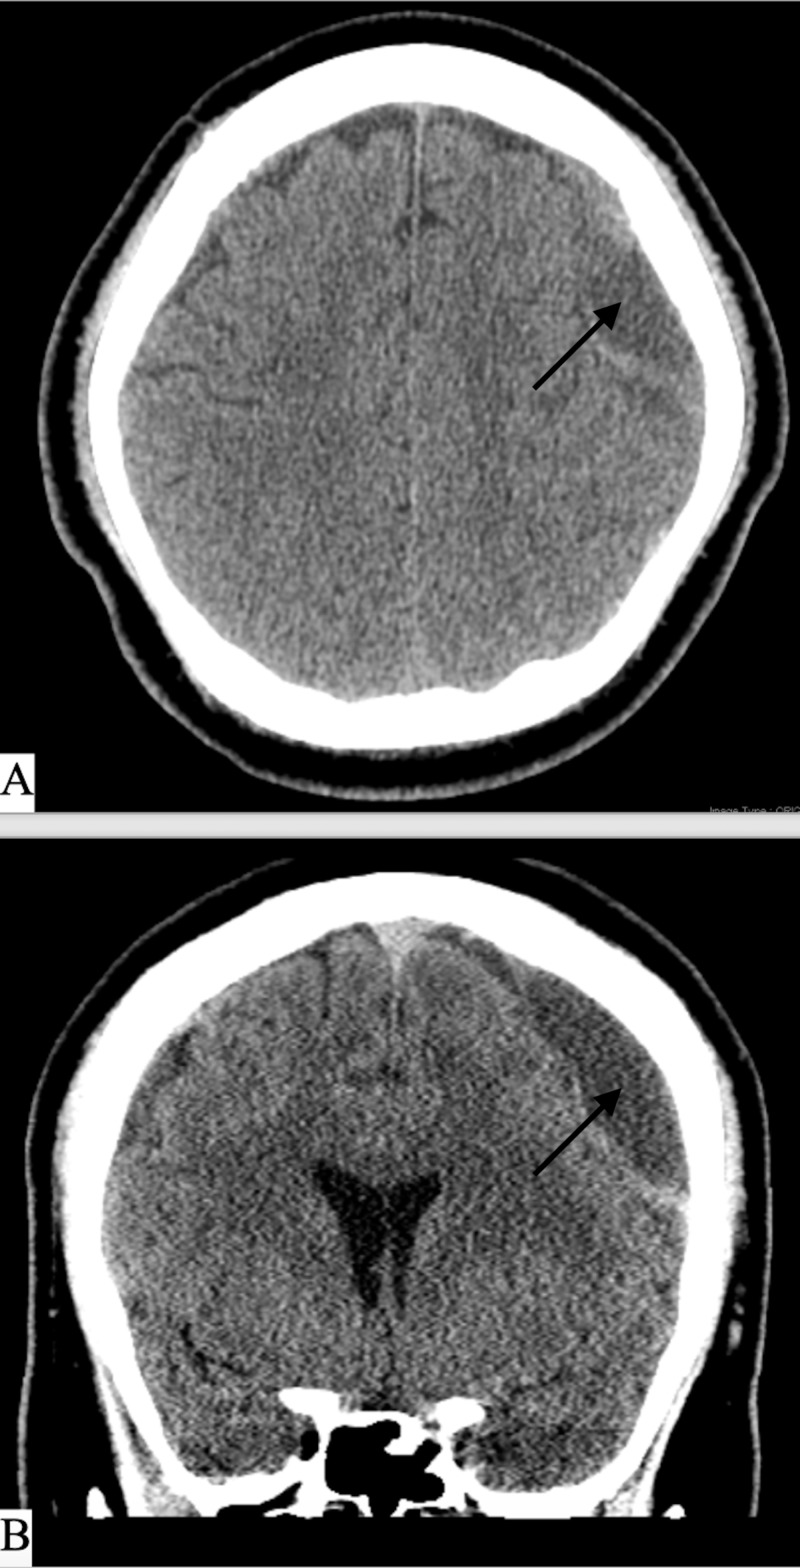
CT head at one-month postop visit A) CT of the head without contrast, axial cut. Obtained at the patient’s one-month follow-up visit. Recurrence of the left-sided subdural fluid collection. B) CT of the head without contrast, coronal cut. Evidence of left hypodense subdural fluid collection; likely hematoma.
